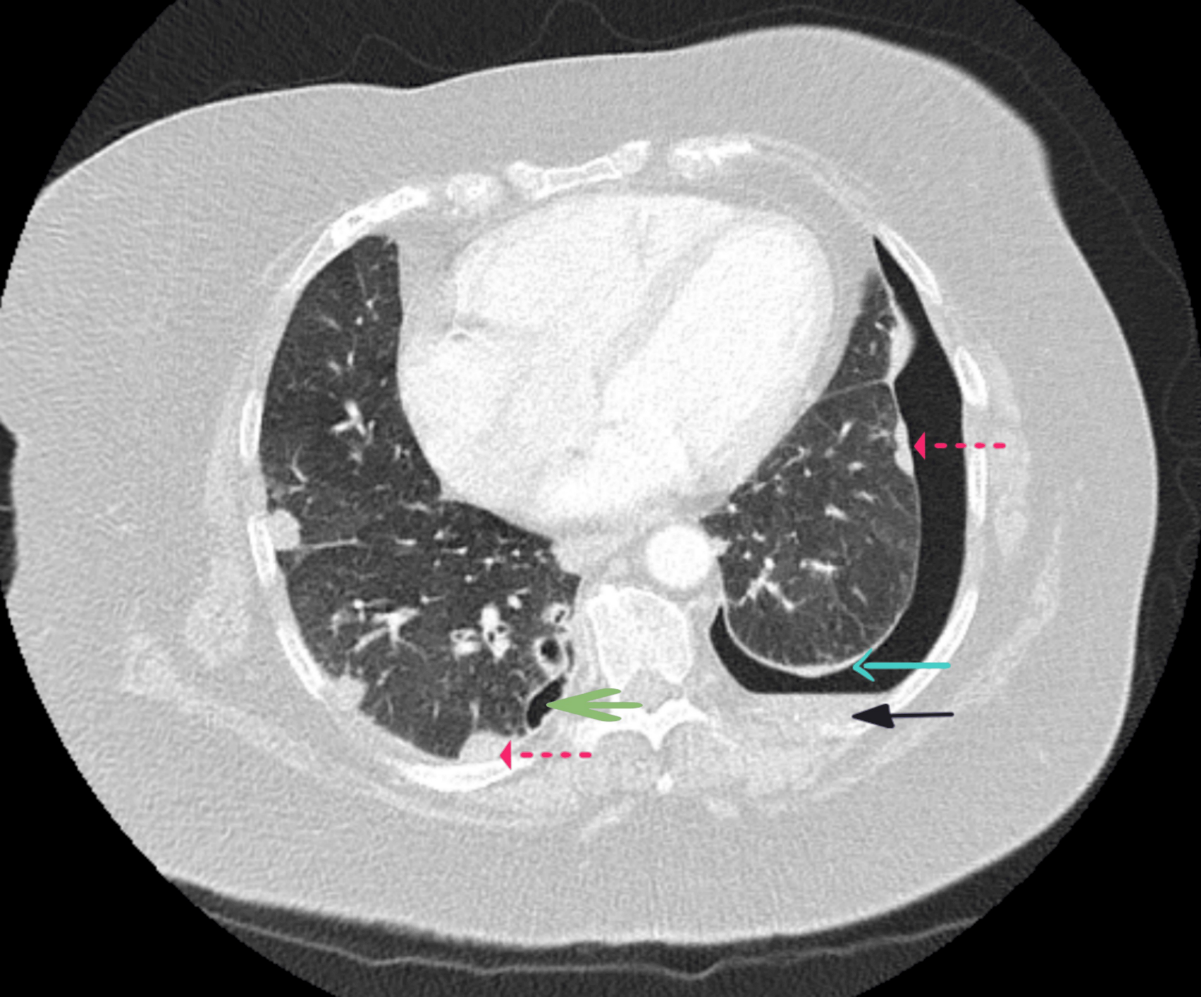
Axial view of contrast-enhanced CT thorax demonstrating a 13 mm left-sided hydropneumothorax, an old loculated right-sided pneumothorax, pleural thickening and subpleural rheumatoid nodules CT: Computed tomography Solid blue arrow: Pleural thickening; Solid black arrow: Pleural effusion; Solid green arrow: Loculated pneumothorax; Dotted red arrow: Rheumatoid nodule
